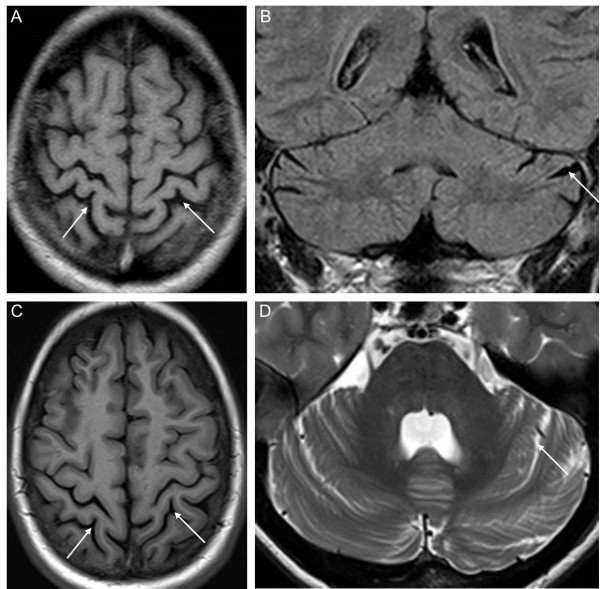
Brain magnetic resonance imaging (MRI) of the two affected siblings. MRI shows diffuse parietal atrophy (arrows in A, C) and mild cerebellar atrophy (arrows in B, D) in the index patient II-2 at age 31 years (upper row) as well as in his sister II-1 at age 38 years (lower row) (A, C T1 axial; B, FLAIR coronar; D T2 axial).
